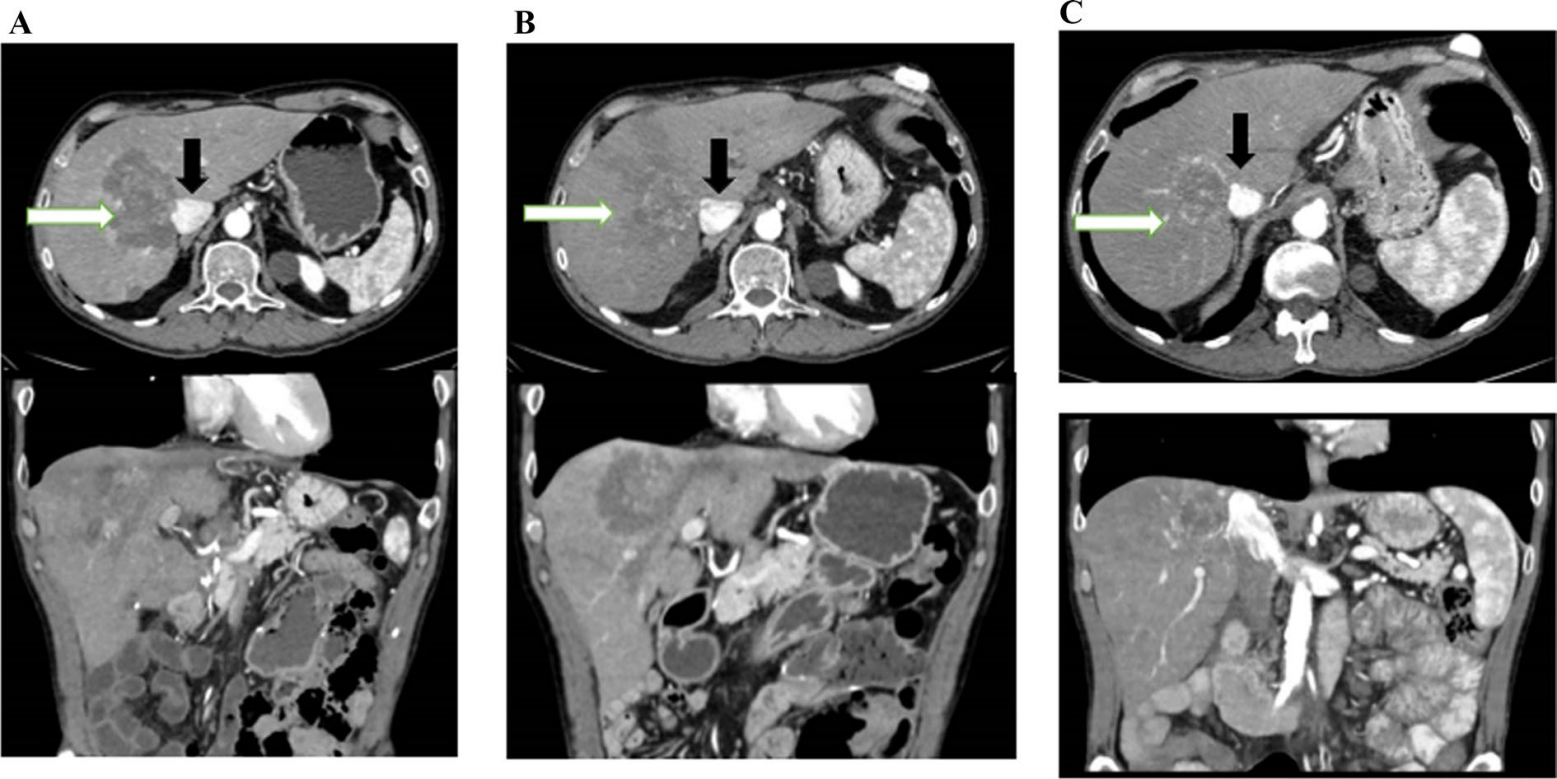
Representative computed tomography (CT) images showing a 60-year-old male patient treated with hepatic artery infusion (HAI) with neoadjuvant intent and after treatment successfully converted to resectable; he was previously treated with 16 cycles of systemic FOLFIRI bevacizumab; white arrow points to lesion, black arrow points to IVC; A before HAI, CT shows unresectable disease with tumor involving the IVC; B 3 months after HAI port insertion treated with HAI oxaliplatin, corresponding 3-month CT demonstrated decreased IVC involvement; C 6 months after HAI port insertion, corresponding 6-month CTs demonstrate continued tumor shrinkage with minimal IVC involvement
